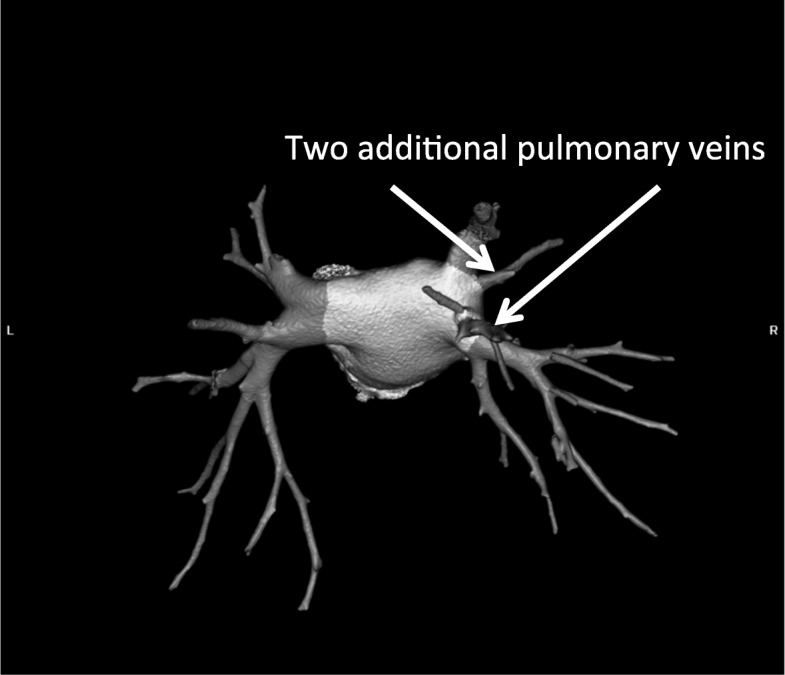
Computed tomography scan with three-dimensional reconstruction of pulmonary veins and the left atrium. The arrow indicates an additional pulmonary vein
